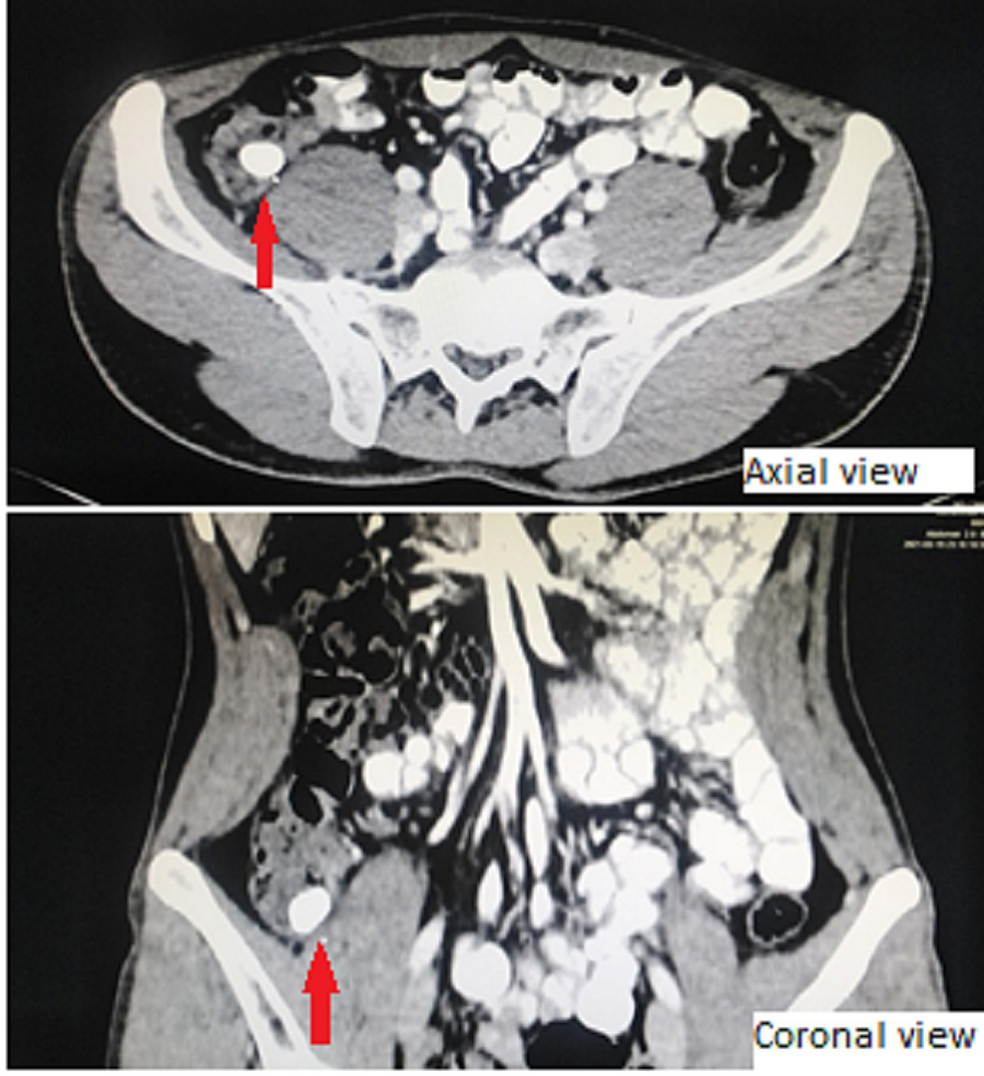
Fecalith as seen on the CT scan
